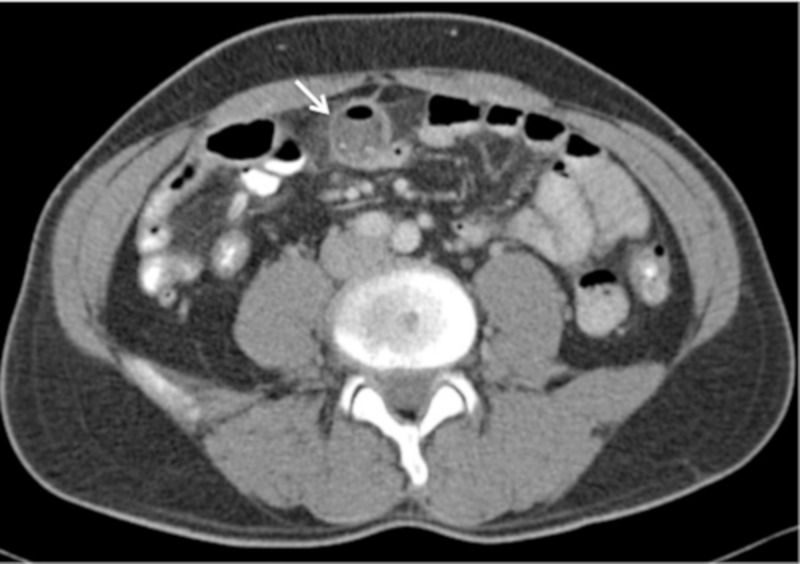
Retrospective review of axial post-IV contrast-enhanced CT imaging revealed a focal out-pouching of the distal ileum (arrow), just inferior and contiguous with the mesenteric collection, containing gas, fluid, and particulate matter with adjacent inflammatory change suggesting a perforated, inflamed Meckel's diverticulum. IV - intravenous, CT - computed tomography
